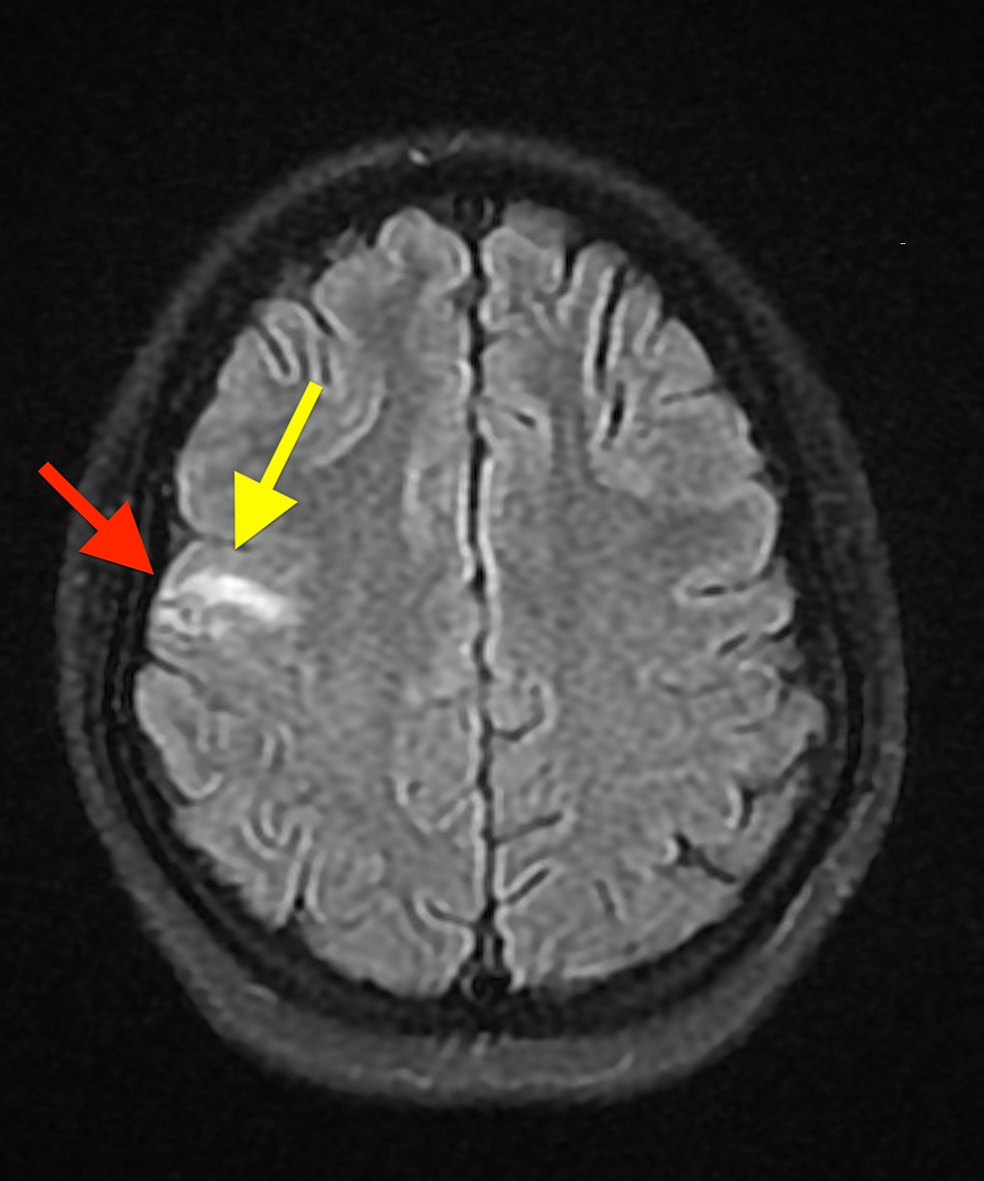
Axial view brain MRI showing peripheral enhancing lesion with an unchanged size (red arrow) and with decreased size of vasogenic edema (yellow arrow).
